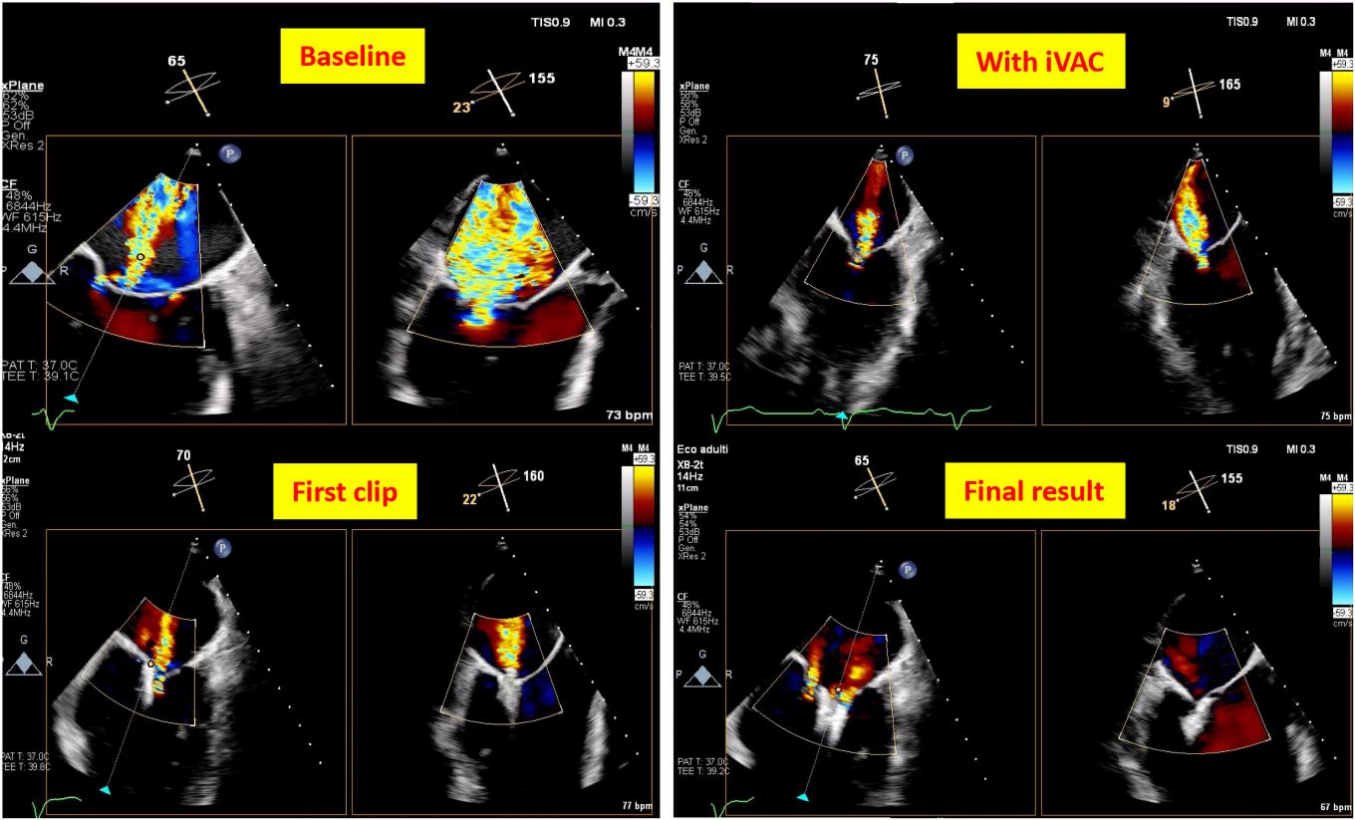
Two-dimensional transesophageal echocardiogram (TEE) with colour flow Doppler during transcatheter edge-to-edge mitral repair showing reduction in the regurgitant jet as the clips are applied and an apparent reduction in LV dimensions with the use of iVAC2L.
